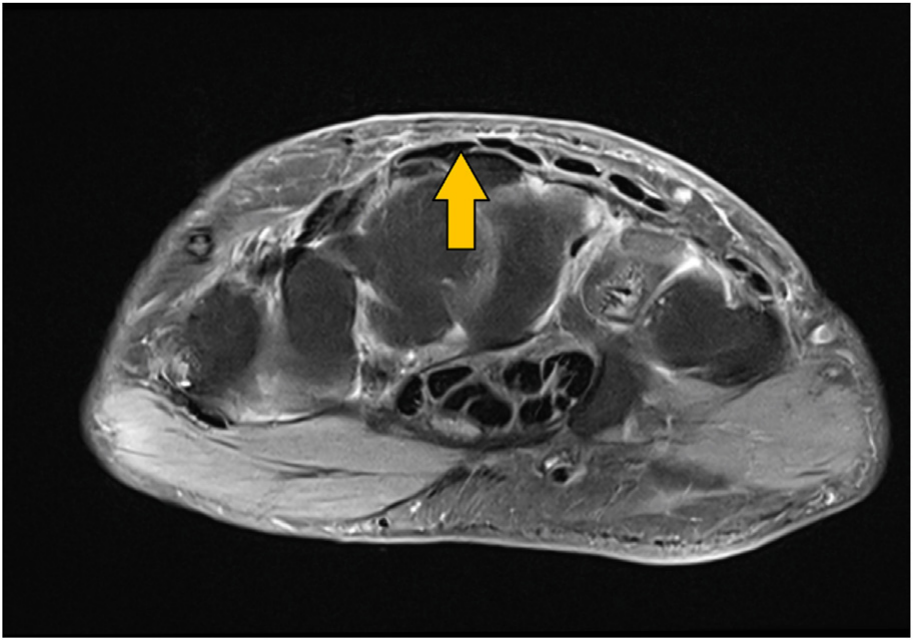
Magnetic resonance imaging of the right hand 8 months after completing chemotherapy showing reduction in dermal mucin (*yellow arrow*).
